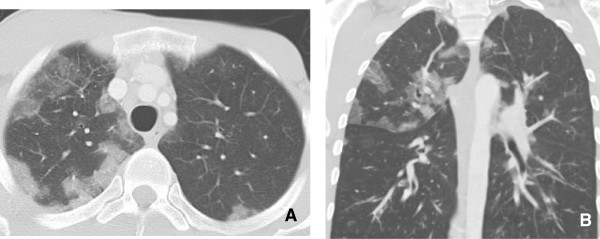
Eosiniphilic pneumonia. A and B. Thin-section (1-mm collimation) CT scan (lung windowing) shows multifocal patchy areas of ground-glass opacity and consolidation predominantly located in the periphery of upper lobes. Findings are consistent with Eosiniphilic pneumonia.
